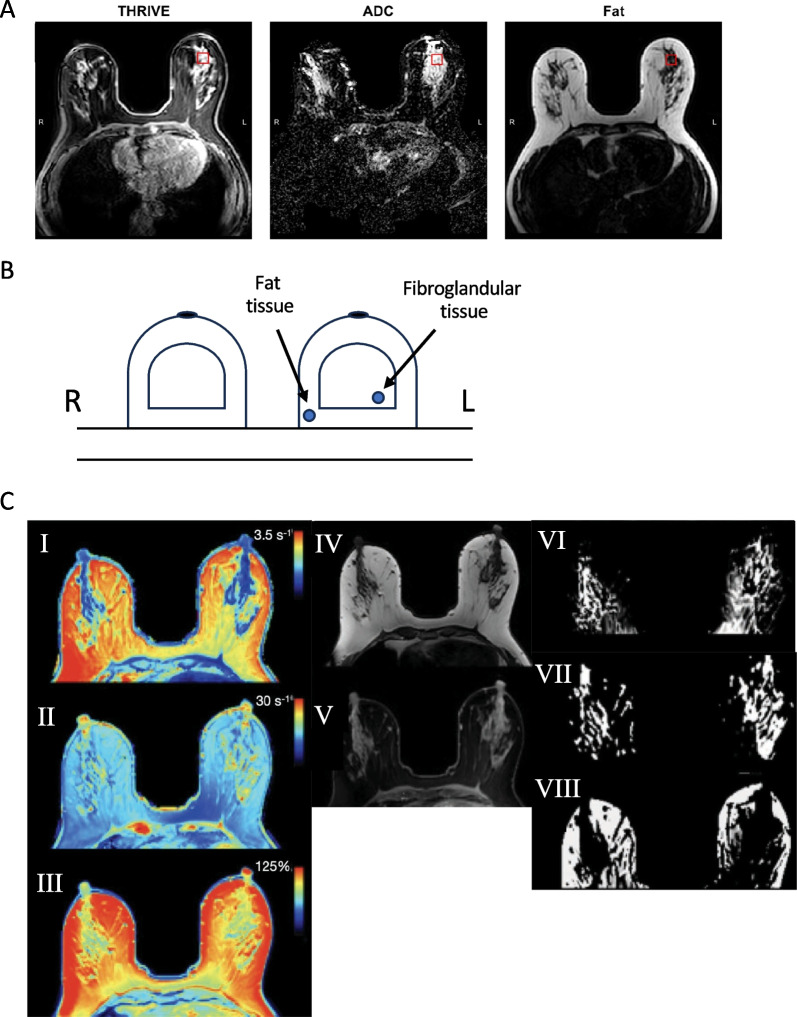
Typical images of multimodal MRI and qMRI maps*.*
